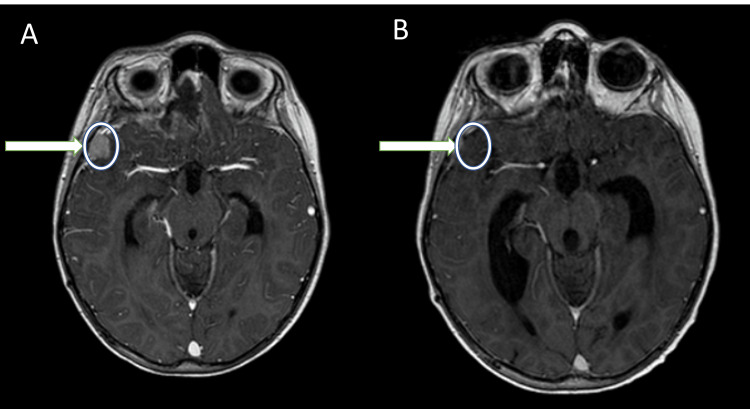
Axial Images of MRI T1WI sequence with contrast at the same level. A: Recurrence of medulloblastoma, the new site at the right temporal pole. B: Three months after focused re-irradiation of the tumour.
